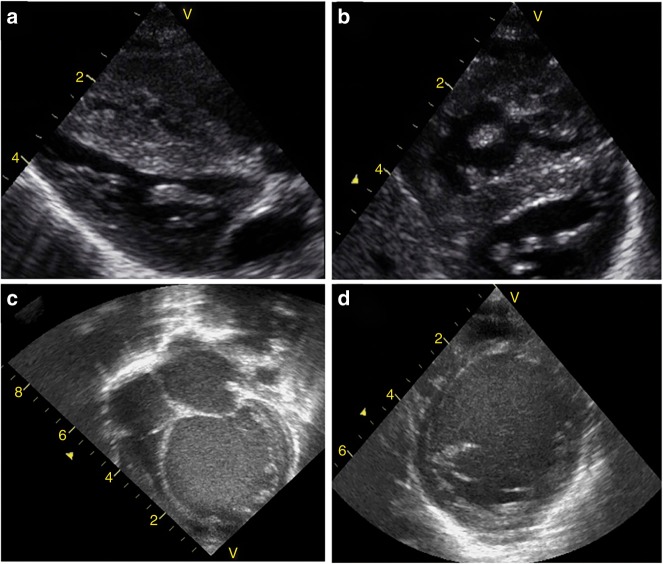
Examples of Echocardiographic findings in common neonatal cardiomyopathies. Infant of diabetic mother: Hypertrophic cardiomyopathy with asymmetric septal hypertrophy. The ventricular walls are hypertrophied, the cavity is small, and ventricular function is normal or hyperkinetic. Two-dimensional echocardiogram showing septal hypertrophy in hypertrophic cardiomyopathy in the parasternal long axis view (Panel a) and the short axis view (Panel b). Arrhythmia-induced neonatal cardiomyopathy (AINC) with severely dilated cardiomyopathy with LV involvement in the apical 4-chamber view (Panel c) and the parasternal short axis view (Panel d)
